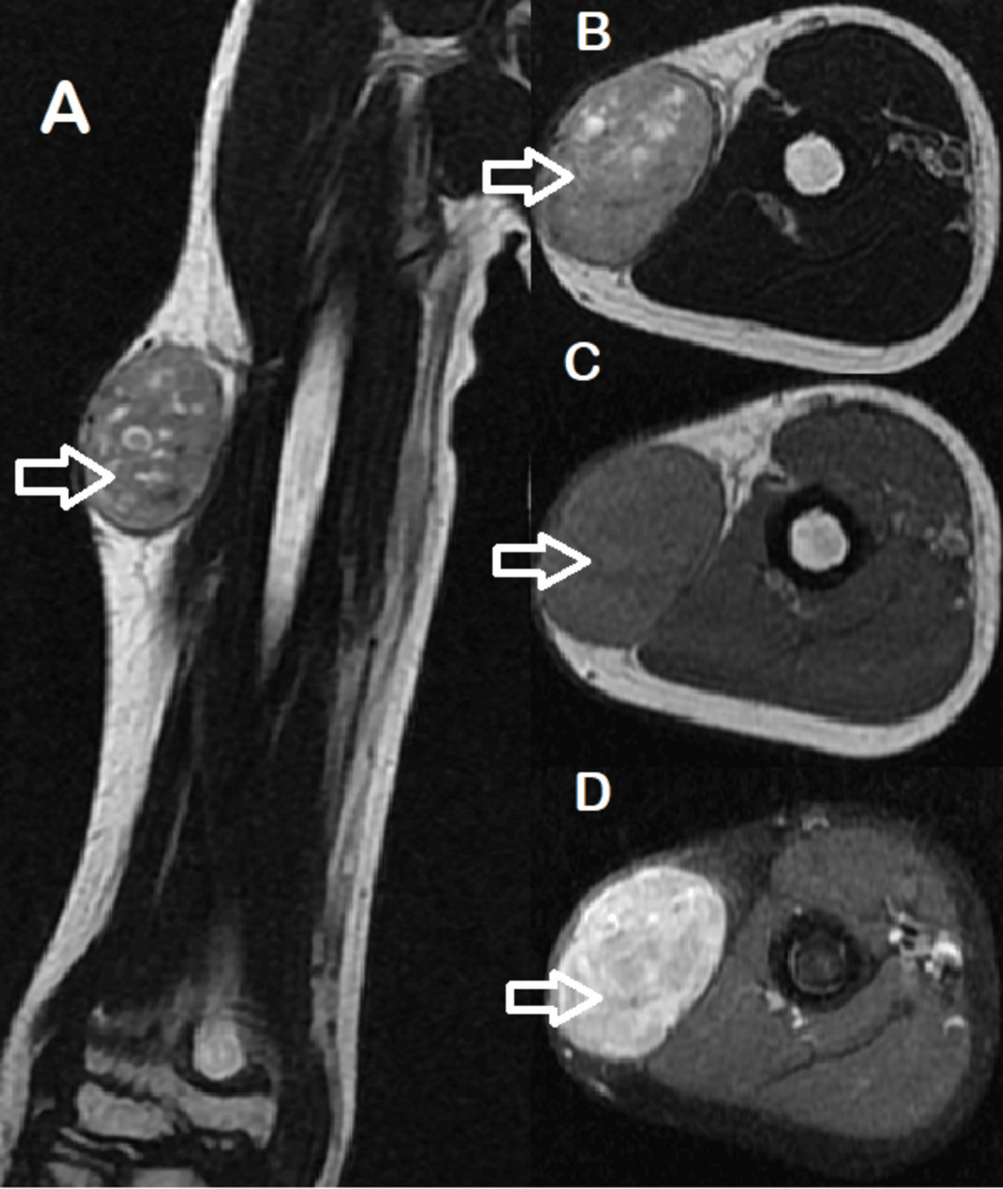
A, B: Coronal and transverse respectivly magnetic resonance imaging slice showing right arm subcutaneous tumoral swelling with regular seams on slight high T2 signal, measuring 67 x 47 mm (white arrow) with a low-signal-intensity capsule. C: Transverse magnetic resonance imaging showing right arm superficial mass exhinbiting heterogenous intermediate signal on T1 (white arrow) with a low-signal-intensity capsule. D: Transverse magnetic resonance imaging showing right arm lateral mass taking uniform contrast (white arrow ).
